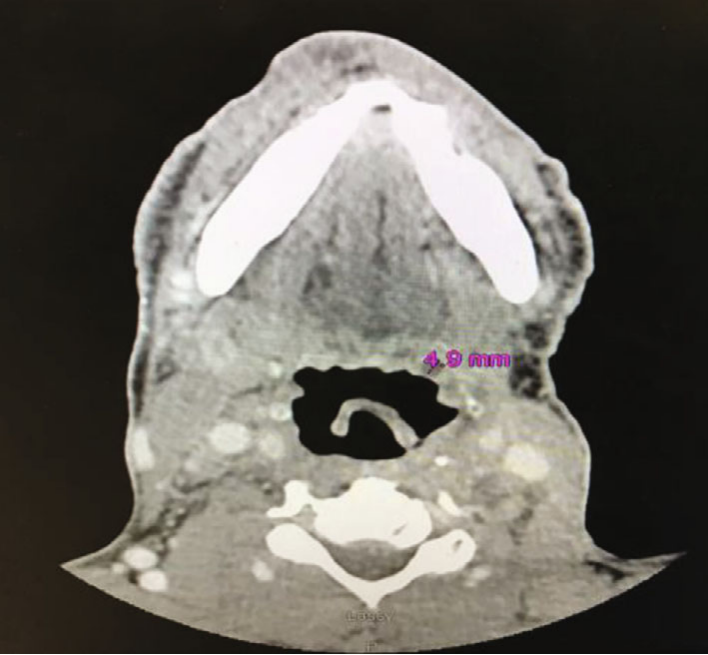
CT neck with contrast imaging of left nasal mass.
